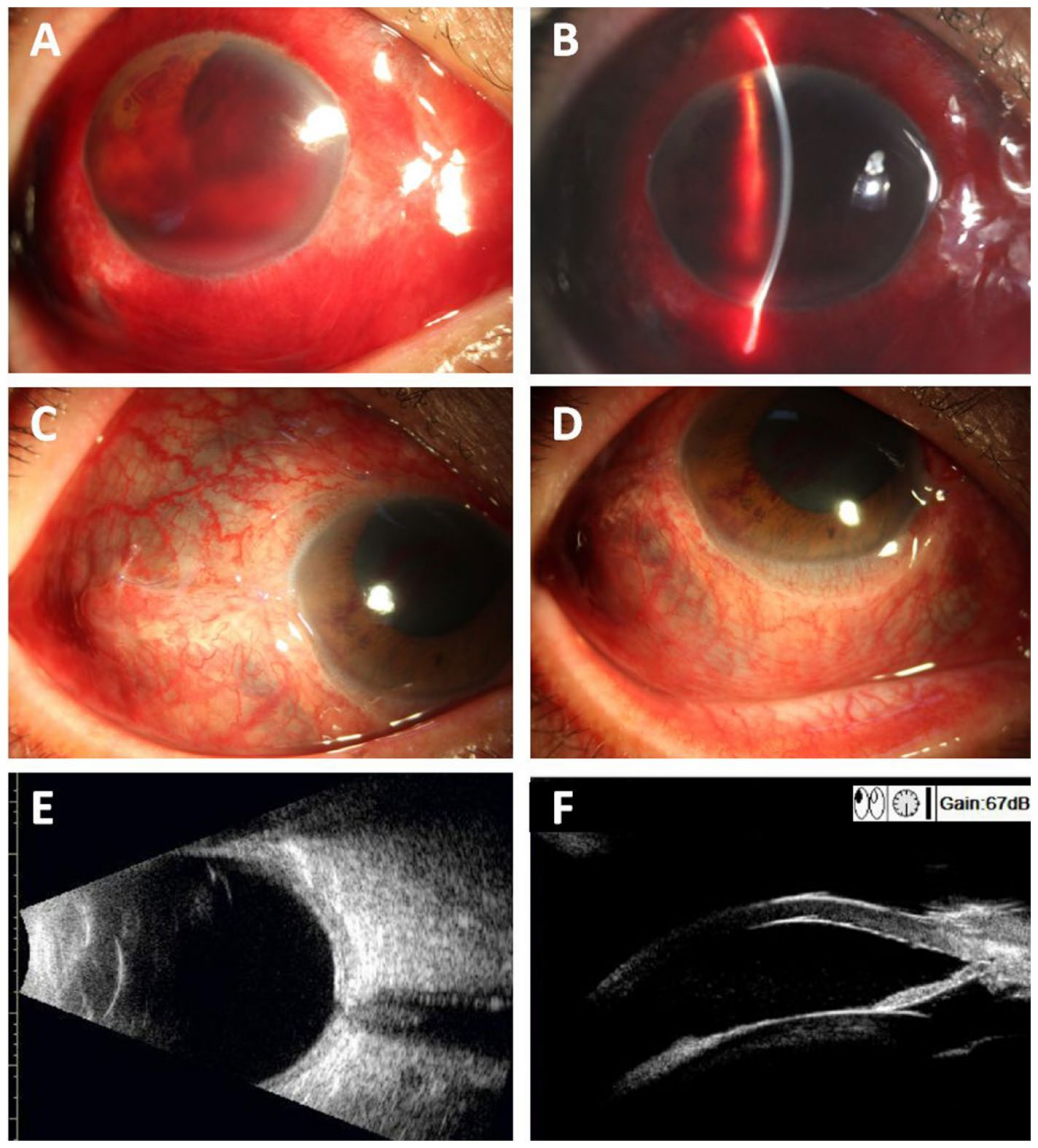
Preoperative photographs of the injured right eye show visible bluish discoloration and scleral thinning. (A,B) Preoperative ocular photographs of the injured right eye (Yellow circles show areas of scleral thinning). (C,D) Postoperative ocular photographs of the right eye 3 weeks after surgery. The yellow arrow indicates the thin sclera with visible subconjunctival uvea. (E) Ocular B-ultrasonography showed mild vitreous opacity in the right eye and slight thickening of the eyeball wall. (F) UBM examination showed an open anterior chamber angle in the right eye.
